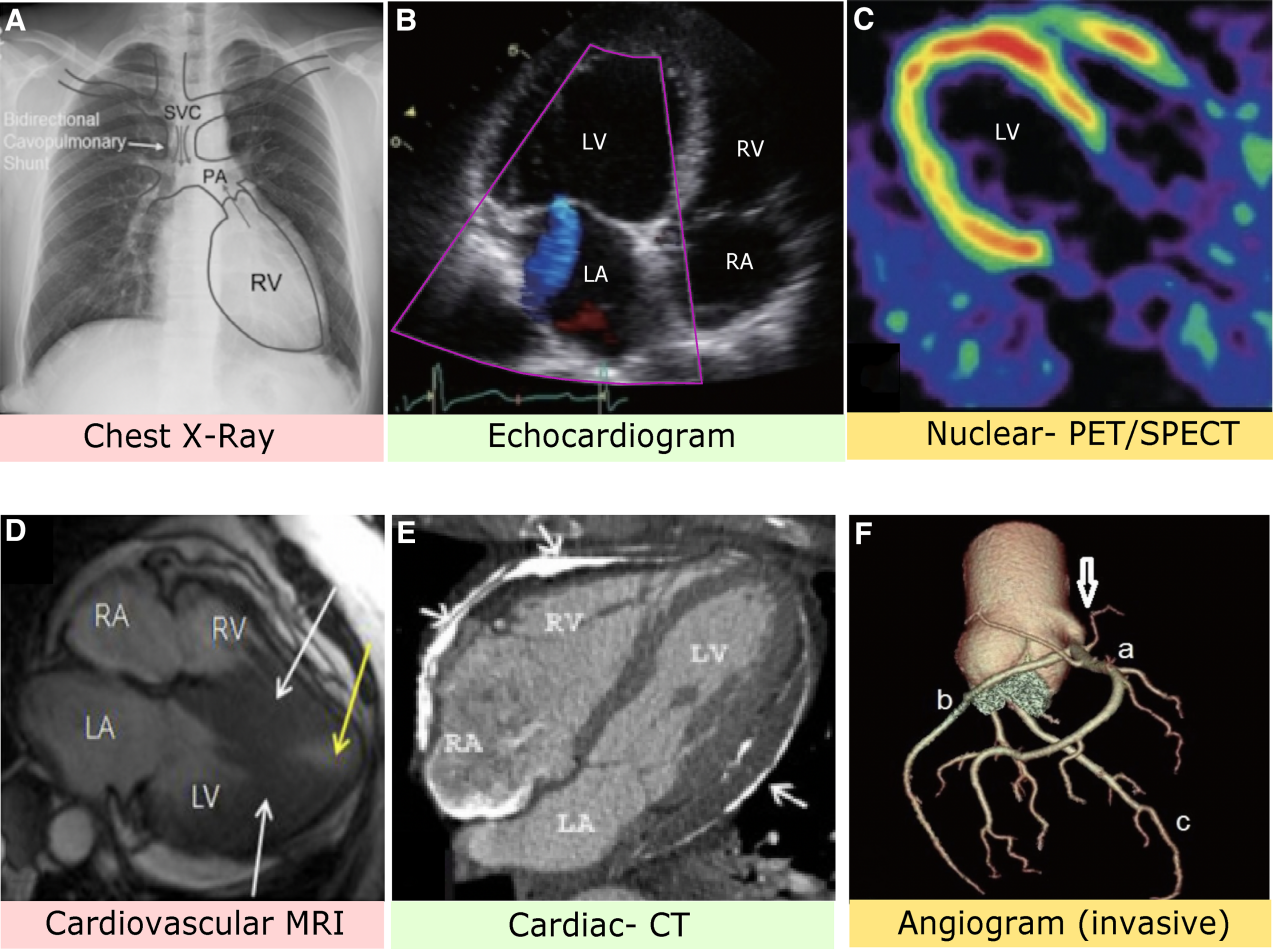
(A) Chest x-ray image with anatomical identification of relevant heart structures. Grey arrows represent blood flow. SVC, superior vena cava; PA, pulmonary artery; RV, right ventricle (8). (B) Stress-induced cardiomyopathy via echocardiogram depicting apical four heart chamber view. LV, left ventricle; LA, left atrium; RV, right ventricle; RA, right atrium (9). (C) PET imaging with contrast allows assessment of blood flow and cardiac sympathetic innervation of the LV (10). (D) MRI with nuclear enhancement revealed an obstructed LV (white arrows) and decreased blood flow. The patient had complaints of angina and dyspnea (11). (E) Axial CT image identifies extensive plaque of pericardial layers (arrows) and deformity suggestive of pericardial constriction (12). (F) Computed CT angiogram indicates the right coronary sinus (arrow) with a mitral valve anomaly. A: Right coronary artery, B: Left circumflex artery, C: Left anterior descending artery (13).
